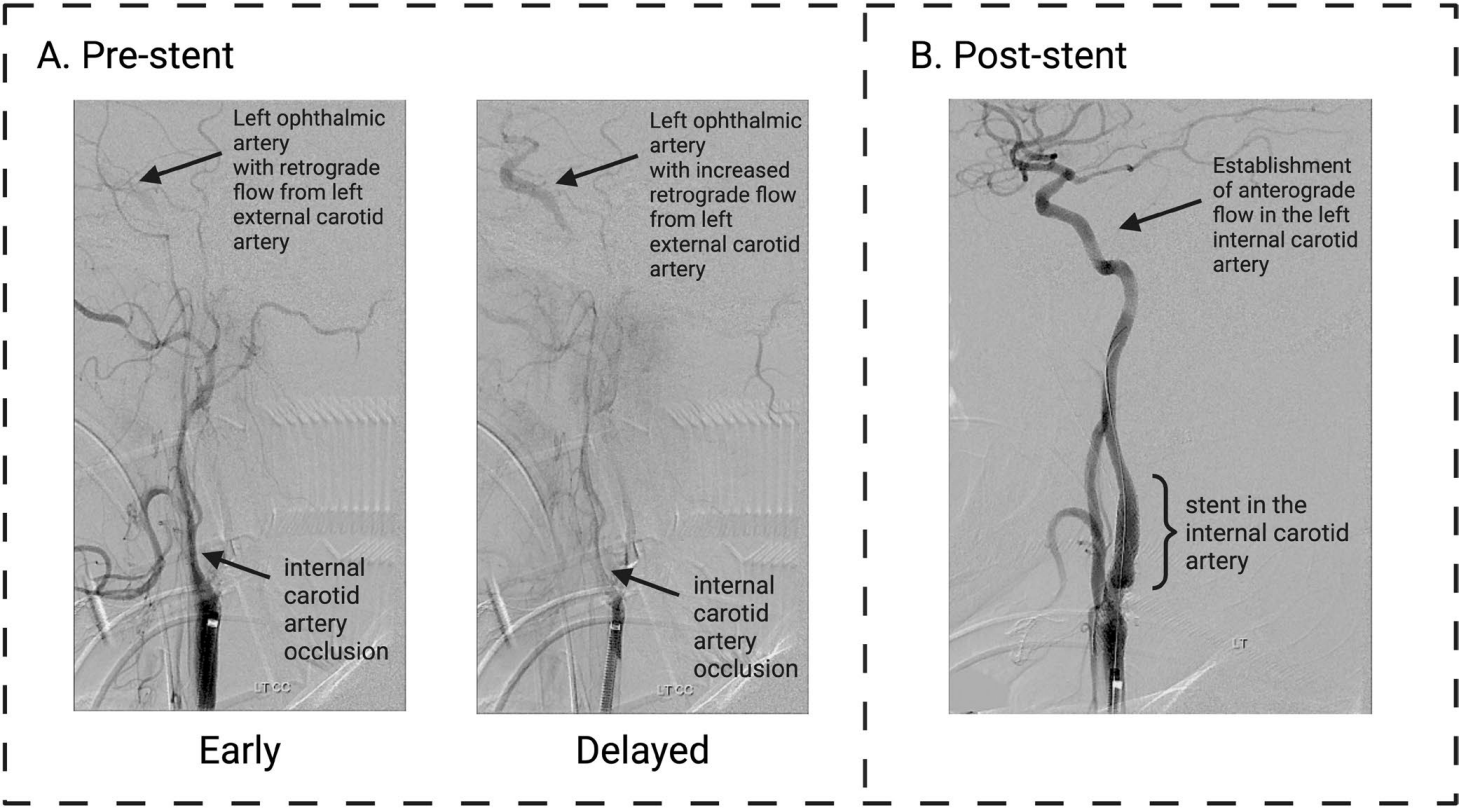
These images show the ophthalmic, external, and internal arteries of the patient before and after the addition of a stent. (A) Shows the computed tomography angiogram identifying the patient's left internal carotid artery occlusion with retrograde flow through the ophthalmic artery. Retrograde flow in the ophthalmic artery increased over time due to the occlusion in the internal carotid artery. (B) Demonstrates resolution of occlusion and return of normal antegrade flow in the internal carotid artery after the addition of a stent.
